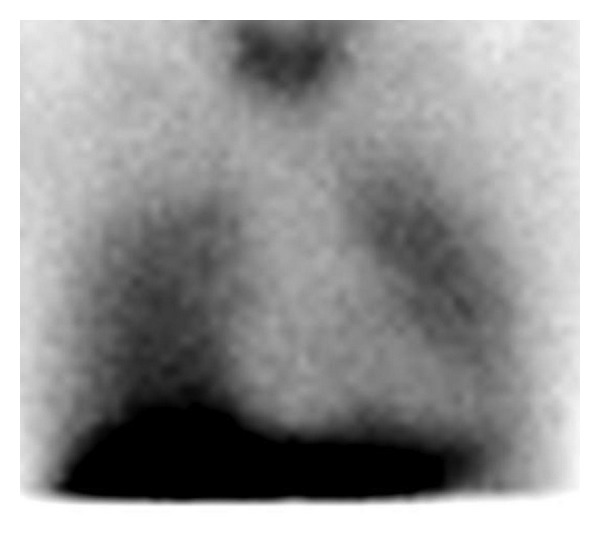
SPECT images showing a decreased myocardial ^123^I-MIBG uptake in the whole left ventricle.
